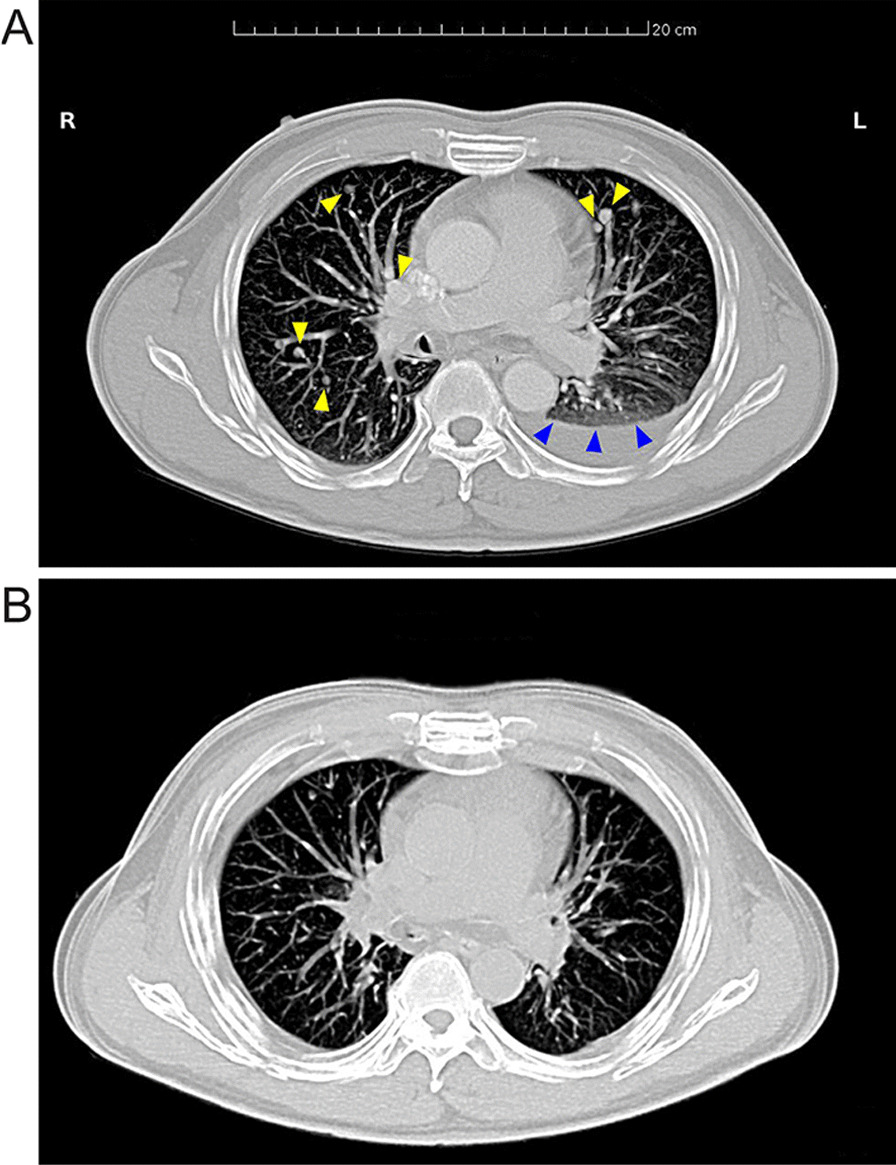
The chest CT images of the patient before and after Gefitinib treatment. A The patient’s chest CT image at the initial diagnosis showed multiple parenchymal tumor nodules (yellow arrows). Lymph nodes were affected as well as the accumulation of effusion fluid in the left pleural cavity (blue arrows). The patient was categorized as a stage c-T2N2M1. B The chest CT image of the patient after receiving Gefitinib treatment for 13 months
